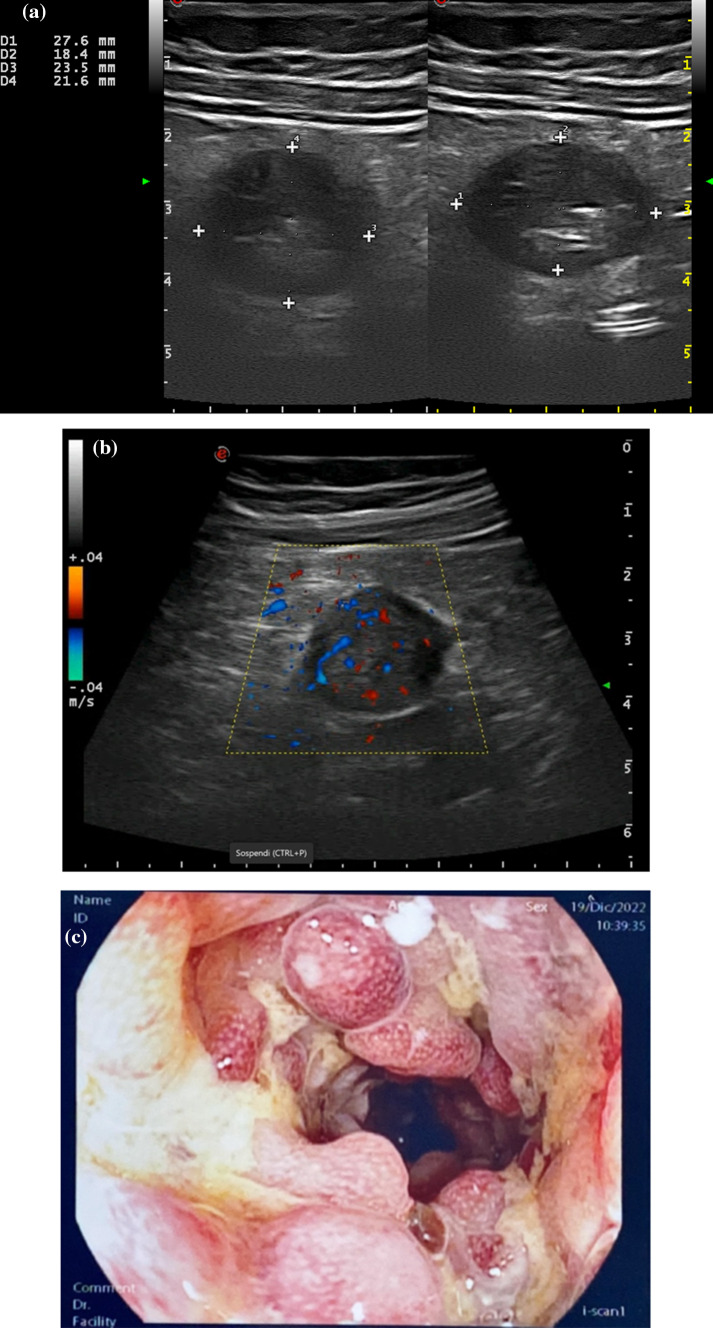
Cross sectional intestinal ultrasound image of the descending colon in a patients with colonic Crohn’s disease in clinical remission showing massive pseudopolyposis as an increased anteroposterior diameter, in two different close segments (a) with color Doppler signals spreading within the lumen (b, Video 1). Endoscopic features of the correspondent colonic segment showing massive pseudopolyposis (b)
